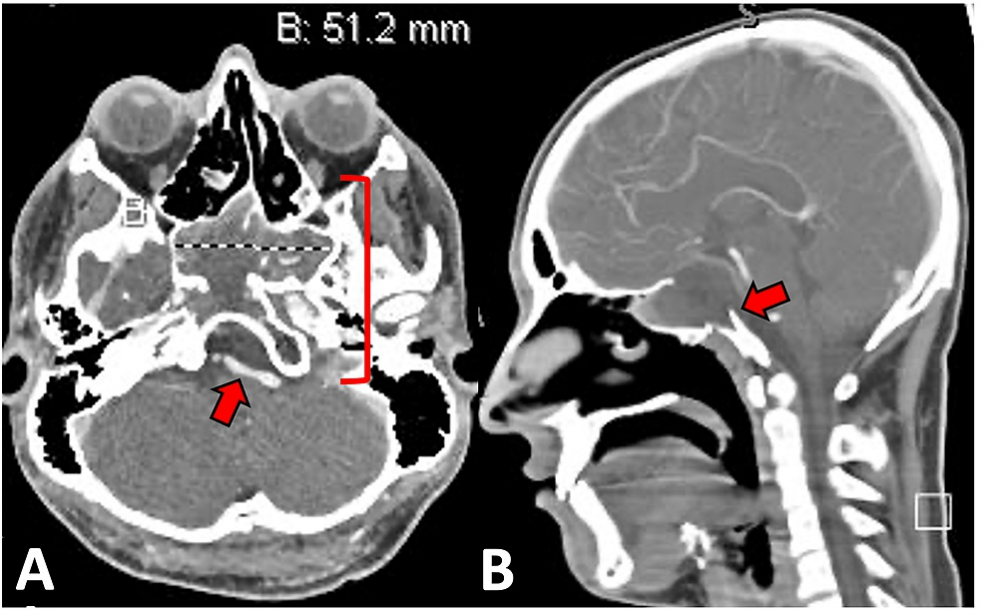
CT angiography Axial (A) and sagittal (B) CT angiogram demonstrate a large 5.1 x 4.7 x 3.0 cm (ML, AP, CC) hypodense lesion. The intratumoral contents contain a predominately homogenous substance of nine Hounsfield units. The lesion is eroding surrounding bone and expanding anteriorly near the sphenoethmoid junction, and inferiorly resulting in a bifid, eroded appearance of the clivus (arrow on image B). The cavernous (C4) and paraclinoid (C5) segments of the internal carotid artery appear as a curvilinear enhancement outlining the tumor margins (bracket on image A). The basilar artery appears as an "i" shaped enhancement anterior to the cerebellum (arrow on image A). ML - medial-lateral; AP - anterior-posterior; CC - cranial-caudal
